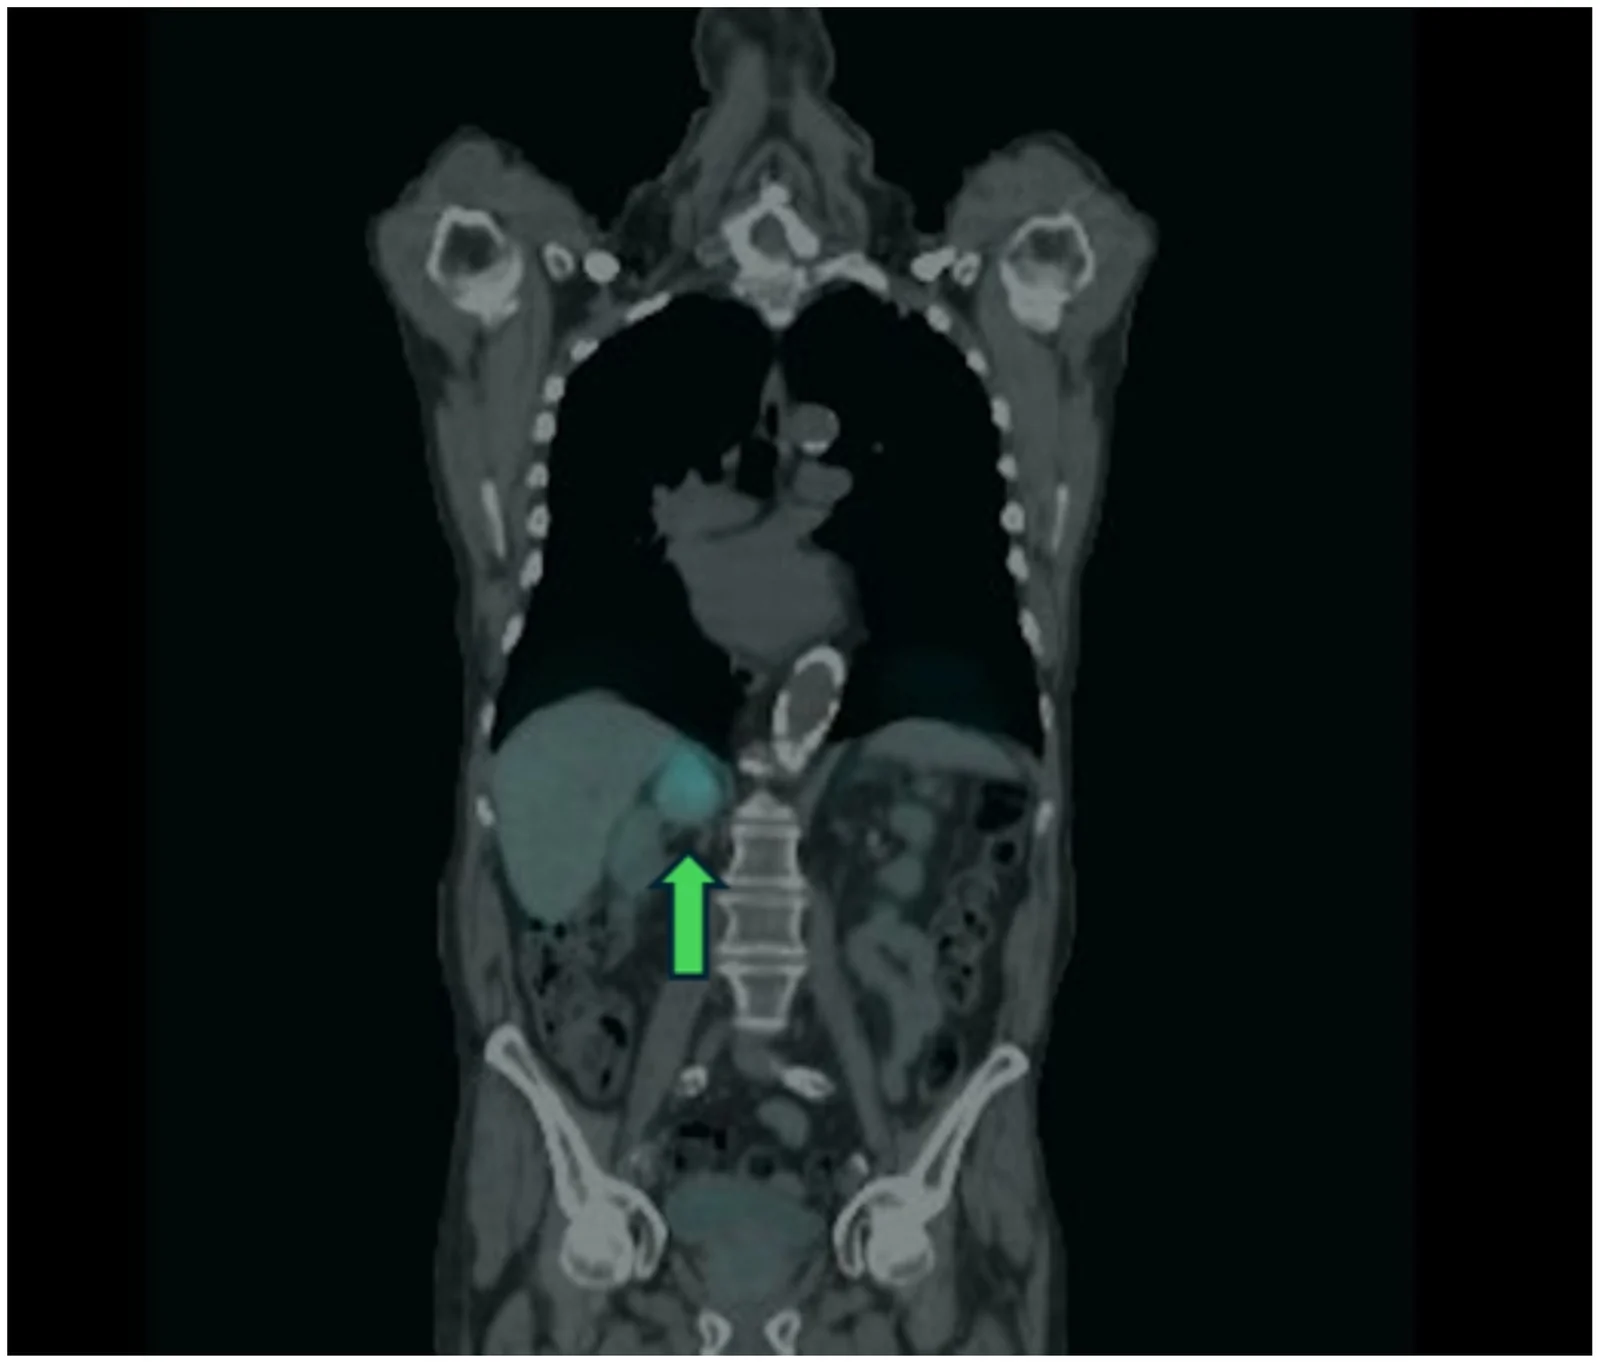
Functional PET/CT evaluation. Coronal ^68^Ga-DOTA-TATE PET image showing increased uptake confined to the right adrenal gland (arrow).
